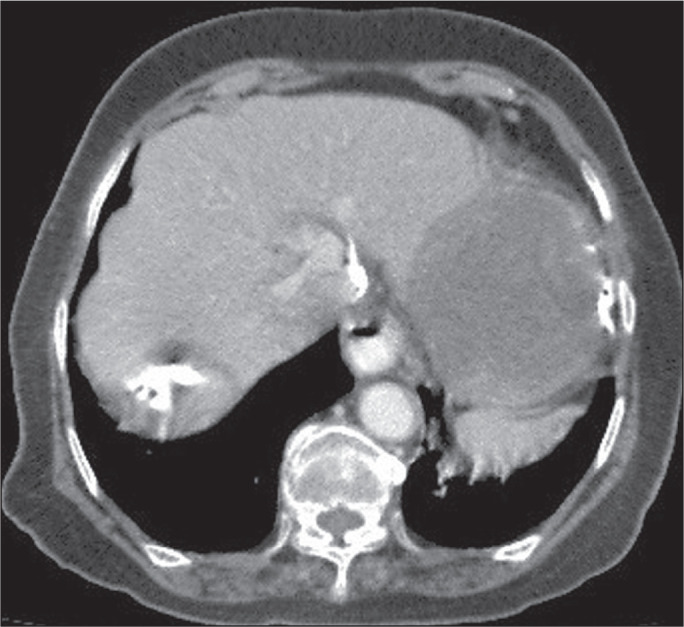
Chest CT scan showing features consistent with hydatid cysts in the left
